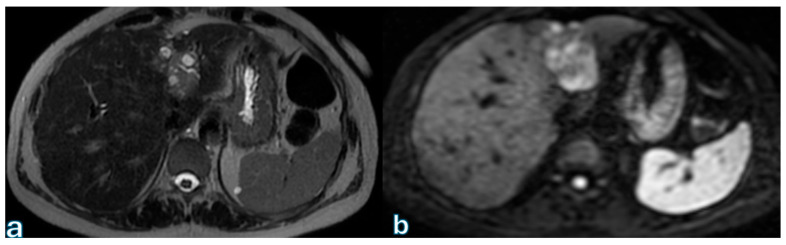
T2-weighted MR (a) and DWI (b) imaging sequences showing a secondary lesion at level IV of the liver segment. This finding was observed in a patient at the third follow-up, conducted approximately 9 months after surgery for pancreatic adenocarcinoma located in the head.
